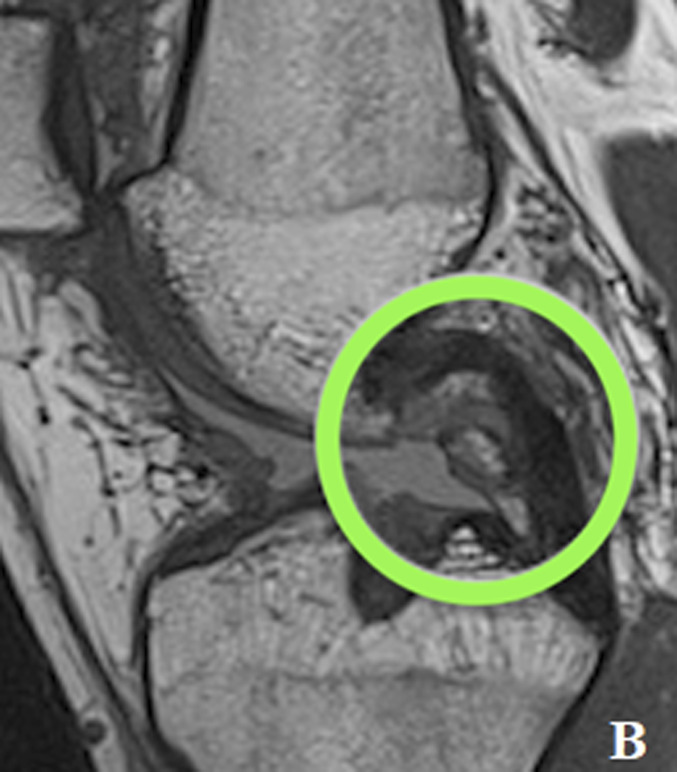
Preoperative knee CT was performed to plan the ACL revision and assess the position of the tunnels and any osteolysis. The CT showed no significant widening of both tunnels. According to Bernard and Hertel's grid^3^, the tibial tunnel was semianatomic, and the femoral tunnel was vertical-anterior in relation to the anatomic footprint (green dot in Fig. 2). The CT also revealed a bone fragment in the intercondylar notch, indicating bony avulsion of the posterior root of the MM (Fig. 3).
